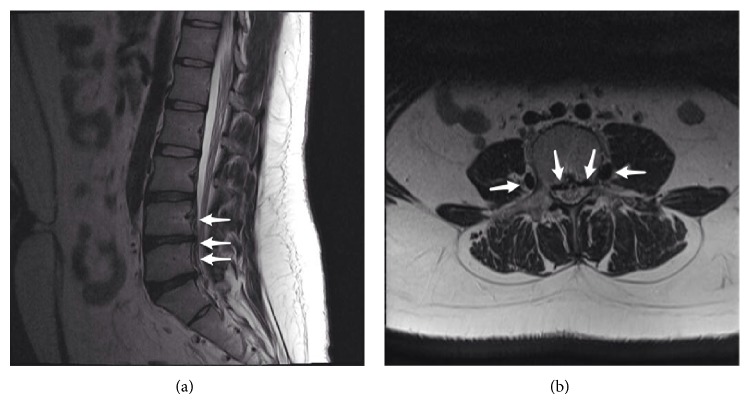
Sagittal T2-weighted image shows vascular enlargement in the epidural area at the level of lower lumbar vertebrae (a). Axial T2-weighted image shows the epidural veins obliterating the epidural space and engorgement of the ascending lumbar vein (b).
